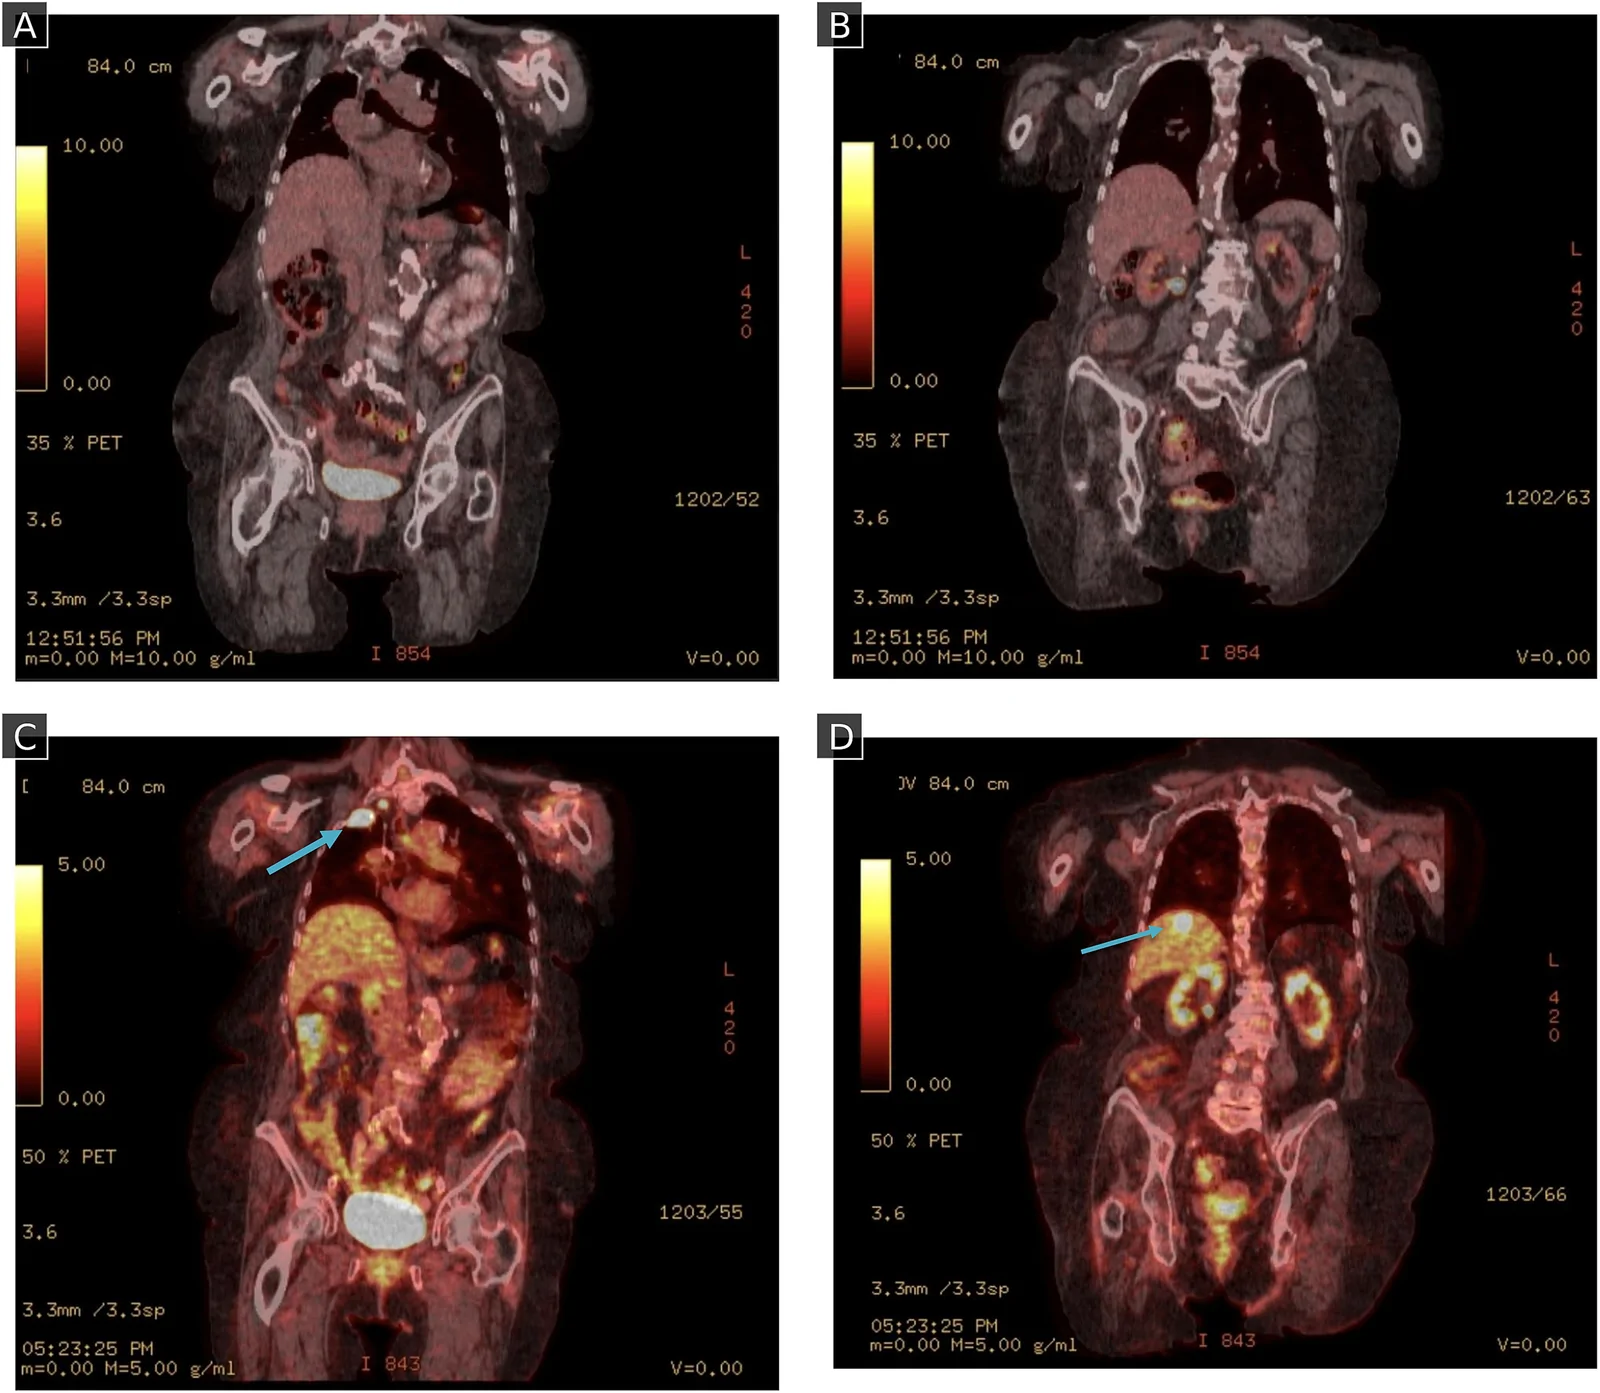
Representative fused coronal PET-CT images before and after nivolumab. Panels C and D are baseline images obtained before pembrolizumab, demonstrating the right apical lung lesion with nodal disease and multiple hypermetabolic right hepatic lesions. Panels A and B are post-nivolumab images demonstrating marked interval metabolic response with resolution of the previously described thoracic and hepatic hypermetabolic disease. Arrows point to the hypermetabolic liver and apical lung lesions.
